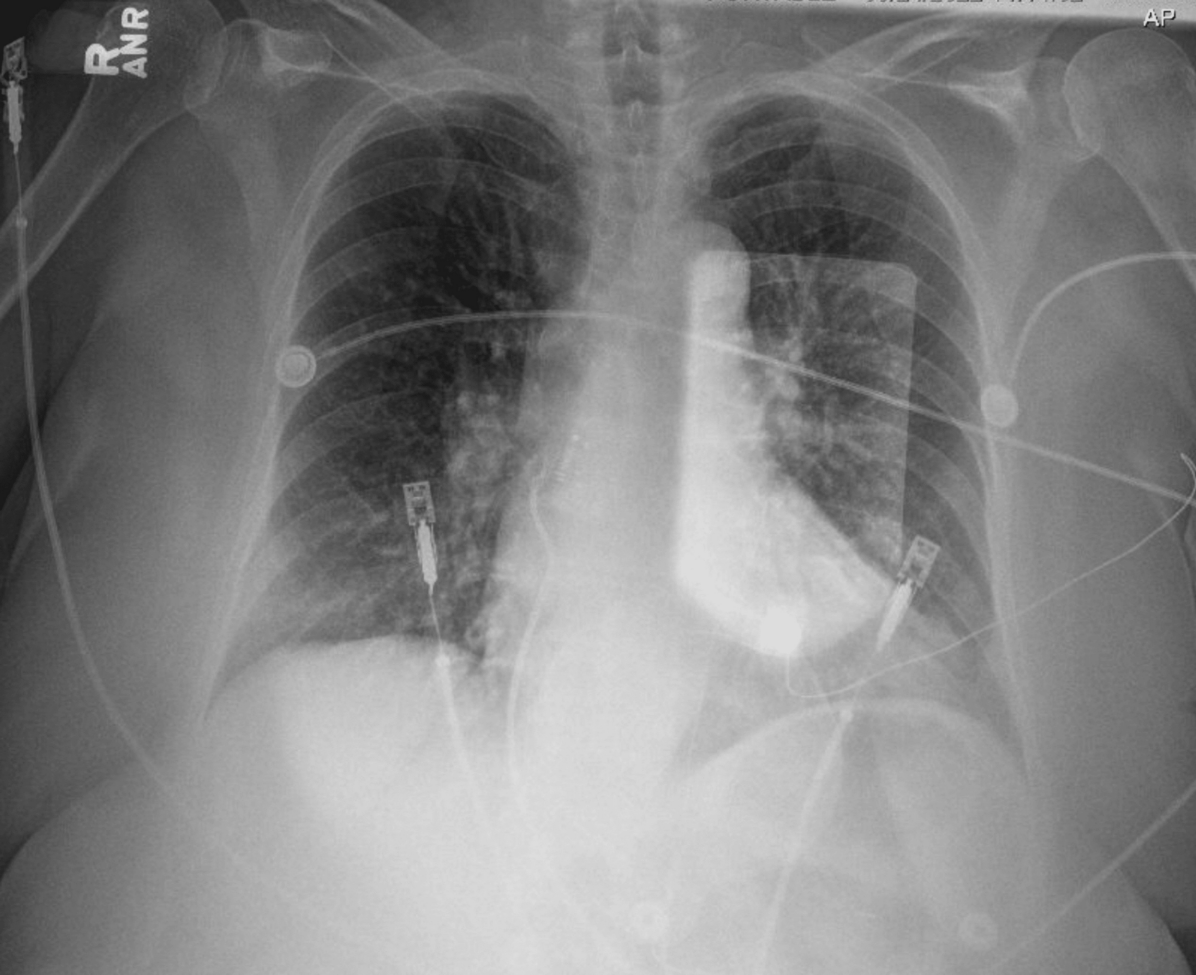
Chest X-ray (CXR) on admission CXR on admission which shows no acute cardiopulmonary findings
